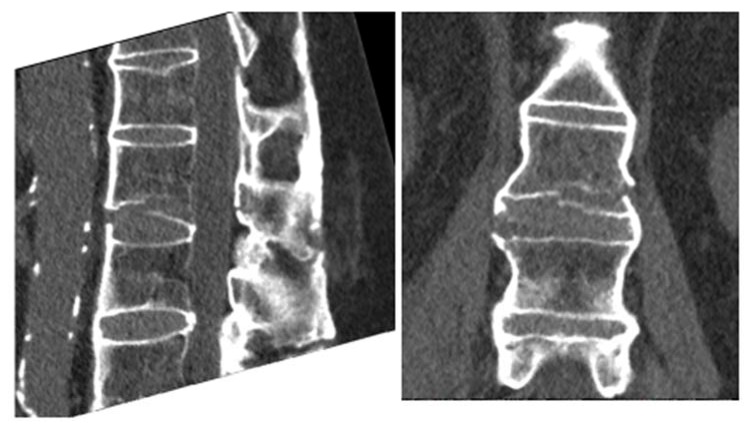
Initial CT scan sagittal and coronal reconstruction.
